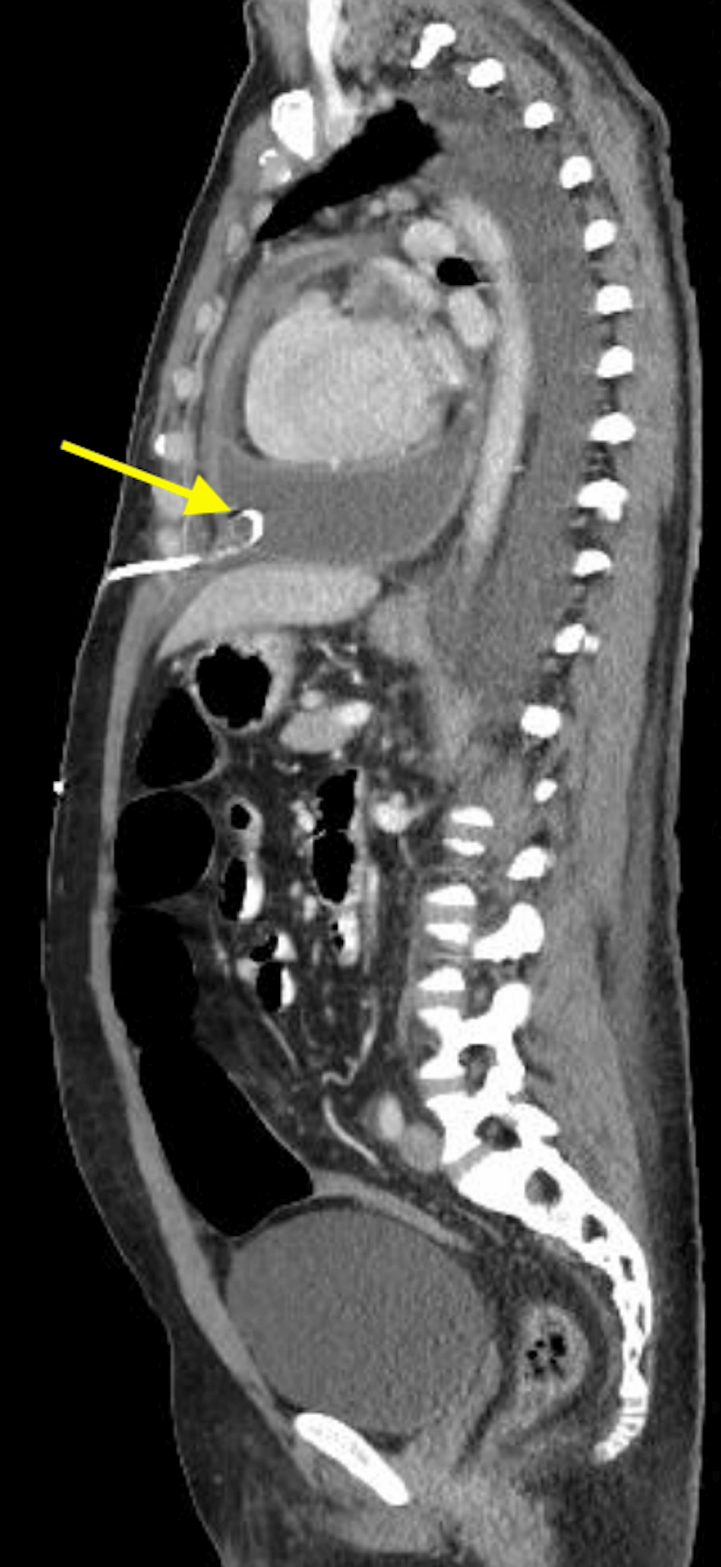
CT scan with contrast showing pericardial drainage catheter in situ in the pericardial space. Yellow arrow pointing to tip of the catheter in pericardial space surrounded by large pericardial effusion.
